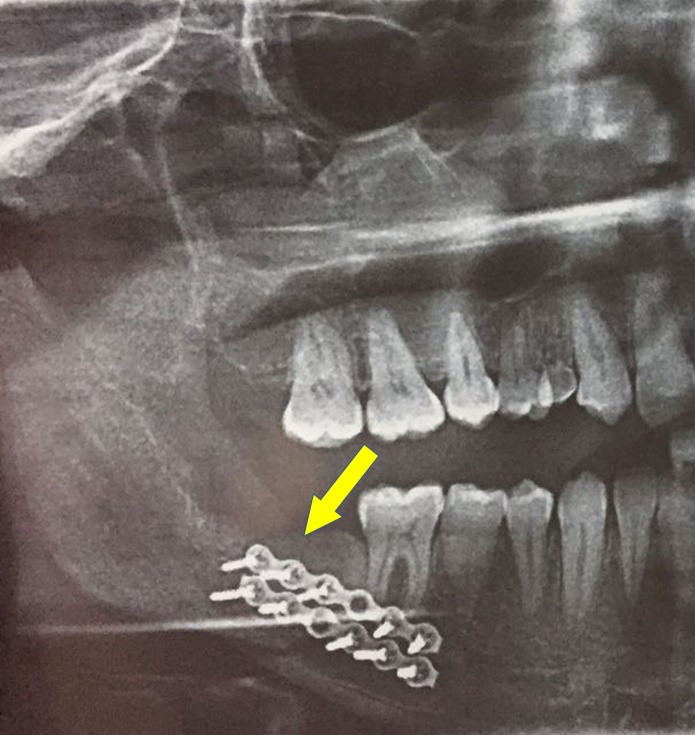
Six months postoperative orthopantomogram showing excellent wound healing
